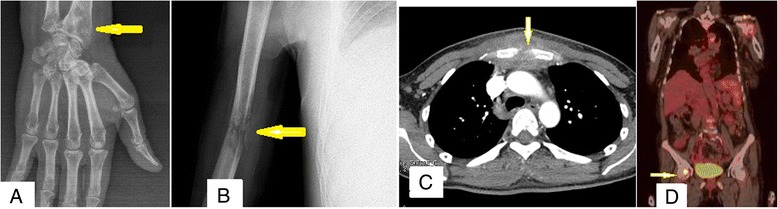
Imaging examinations. Multiple spots or patchy farfetched like bone destruction, periosteal proliferation, fracture, and swelling in surrounding soft tissue was shown *via* radiography a & b, emission computed tomography, high resolution computed tomography c, and positron emission tomography/computed tomography d
